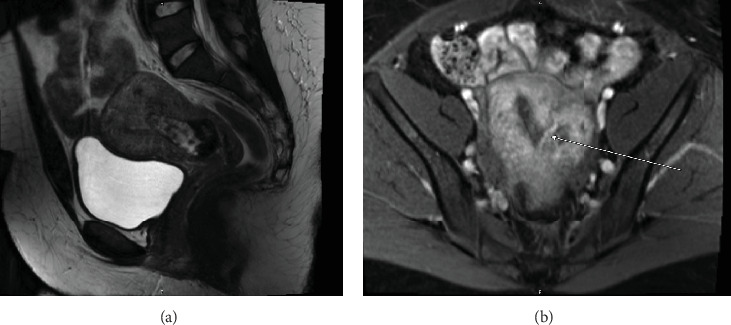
MRI pelvis with contrast. (a) Sagittal and (b) axial views demonstrating a 4-cm intrauterine mass with feeding vessels and areas of acute hemorrhage and hematoma.
